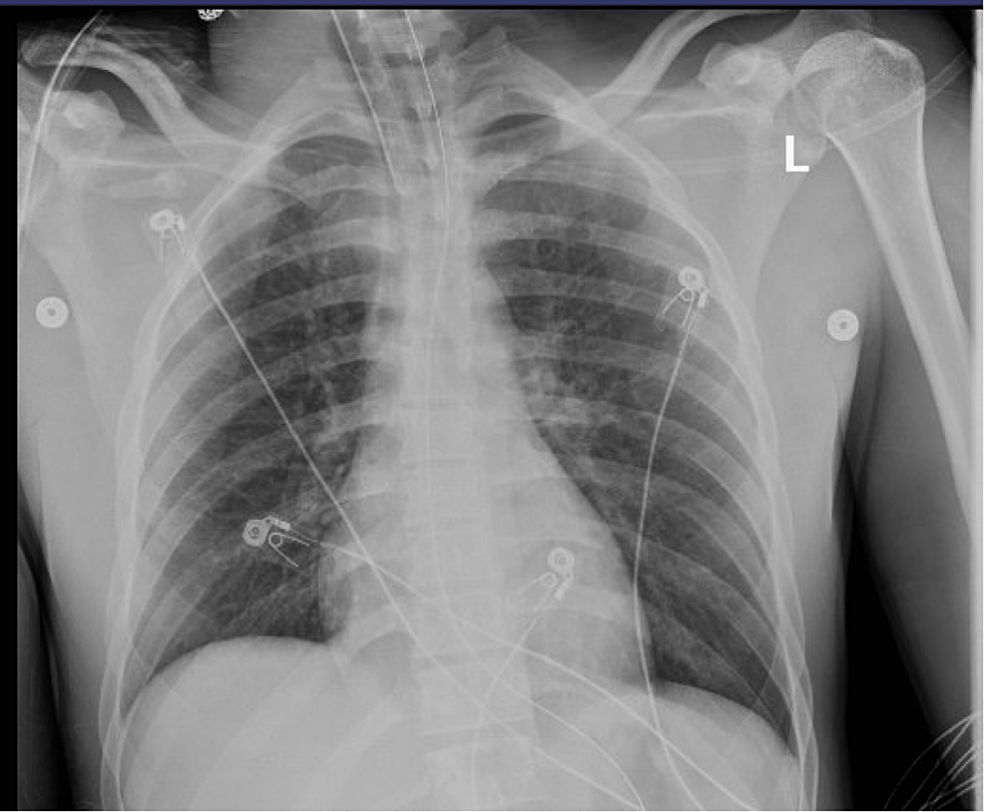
Chest X-ray showed near complete resolution of infiltrates on day three.
